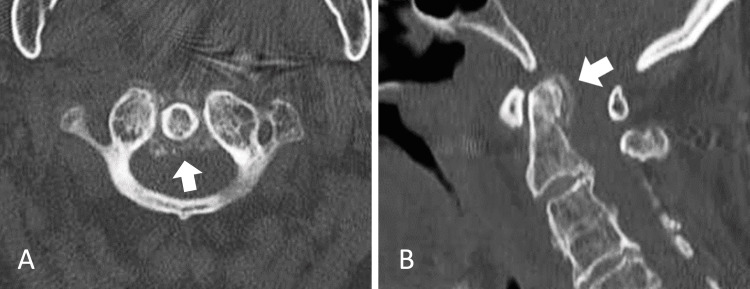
Calcification around the odontoid process on cervical spine CT. A: horizontal section B: sagittal section.
